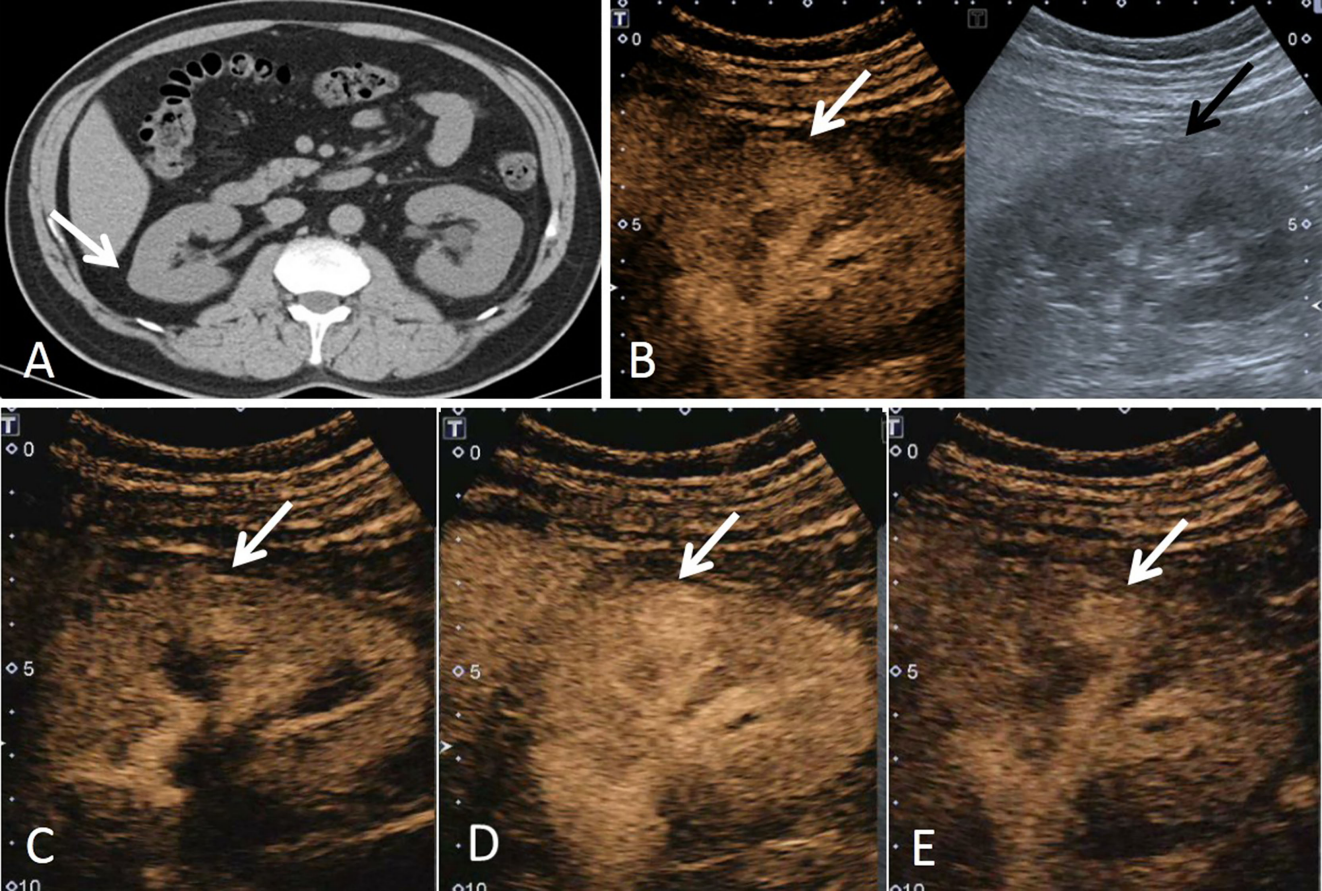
A 43-year-old man had a ccRCC in the right kidney with the size of 1.6 × 1.5 cm. (A) Non-enhanced CT showed a suspicious lesion in the middle of the right kidney (white arrow). (B) According to the position displayed on CT, the simultaneous display mode of conventional ultrasound (CUS, right) and contrast-enhanced ultrasound (CEUS, left) was performed, and CEUS showed a lesion with hyperenhancement (white arrow), but it was not detected on CUS (black arrow). (C) On CEUS imaging, it showed that the contrast agent entered the mass synchronously with the adjacent renal cortex (white arrow). (D) At the peak of enhancement intensity, it showed homogeneous hyperenhancement compared with that of the renal cortex (white arrow). (E) When the contrast agent discharged from the mass, it showed a slow-out pattern compared to the adjacent cortex (white arrow).
